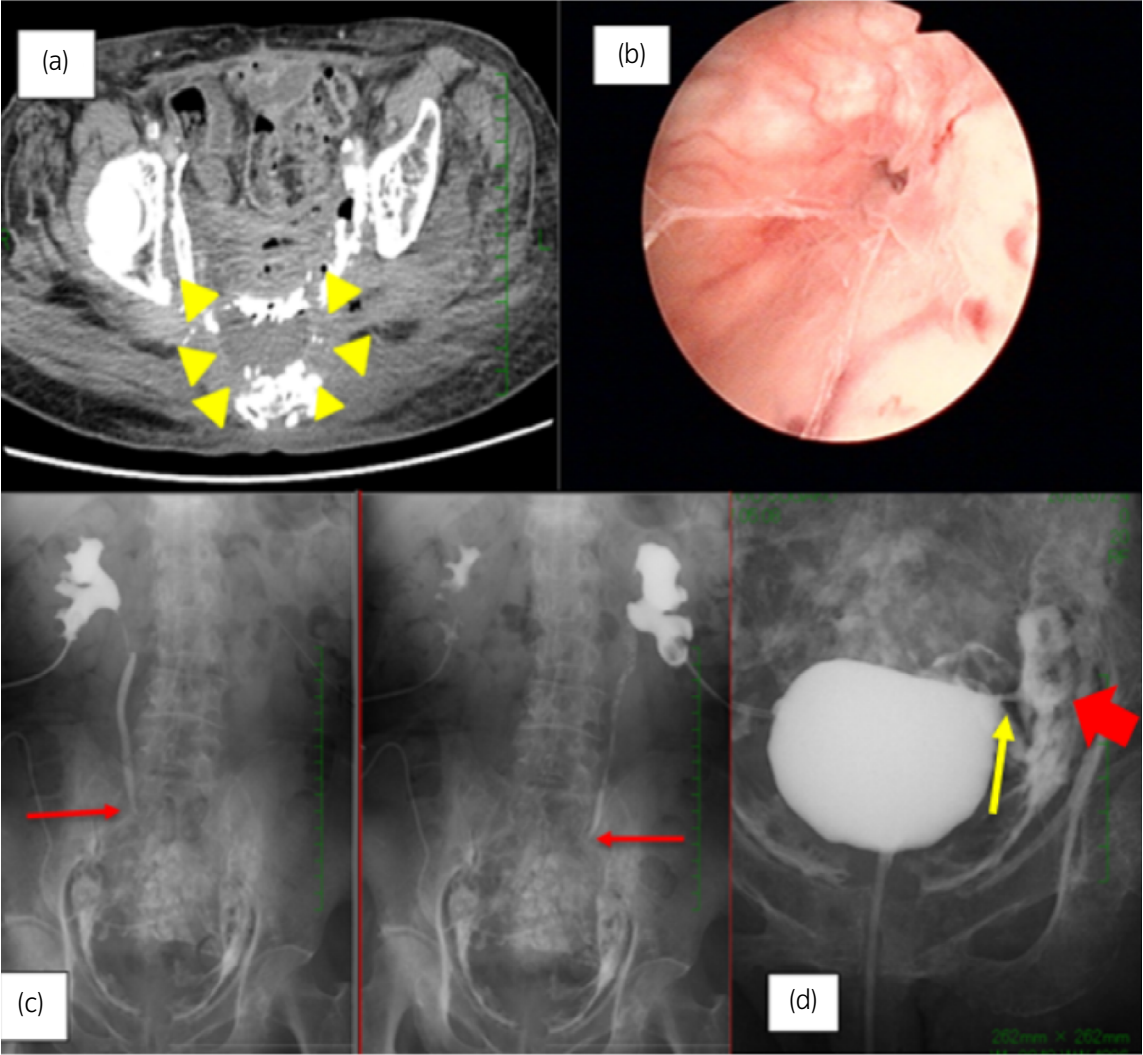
(a) Contrast‐enhanced CT scan identifying liquid in the ventral of the patient's sacrum bone (yellow arrowheads) and calcification around the liquid. This suggests calcification is due to radiation. (b) Cystoscope image indicating a fistula of about 2 mm at the left cyst wall. (c) Antegrade pyelography showing we cannot confirm the contrast under both sides of the mid‐ ureters and in her bladder (red arrow). (d) Cystography showing urine leakage from her left cyst wall (yellow arrow) to the abscess cavity (red arrow).
